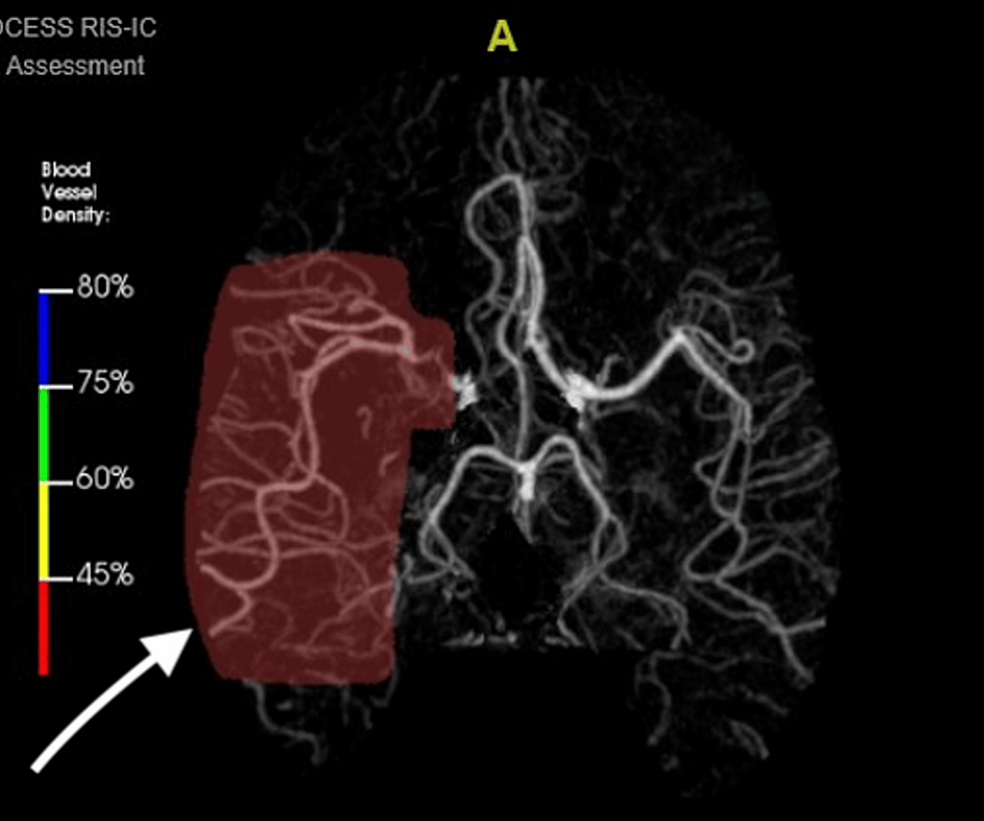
CT angiogram of the brain. Severe stenosis or proximal occlusion of the right A1 anterior cerebral artery segment. Morphology of the occlusive findings suggests chronicity and raises the possibility for vasculopathy such as moyamoya.
